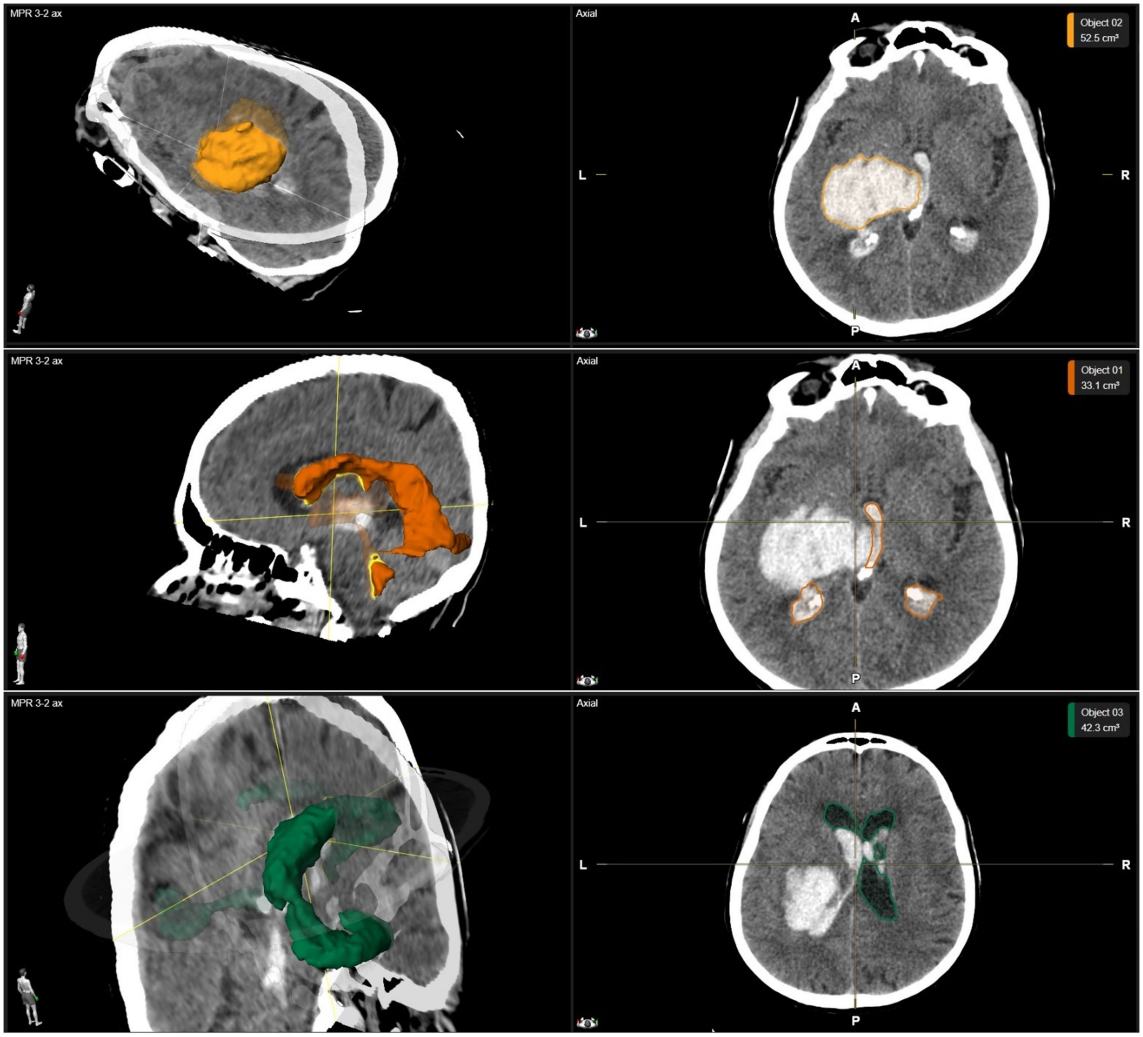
Example of volumetric data. The volumetric analysis of a thalamic ICH with IVH is shown in a three-dimensional reconstruction as well as axial planes of CCT images. Above row: The volume of a thalamic ICH is highlighted in yellow. Middle row: The volume of IVH is highlighted in orange. Below row: The volume of intraventricular CSF is highlighted in green. CCT, cerebral computed tomography; CSF, cerebrospinal fluid; ICH, intracerebral hemorrhage; IVH, intraventricular hemorrhage.
